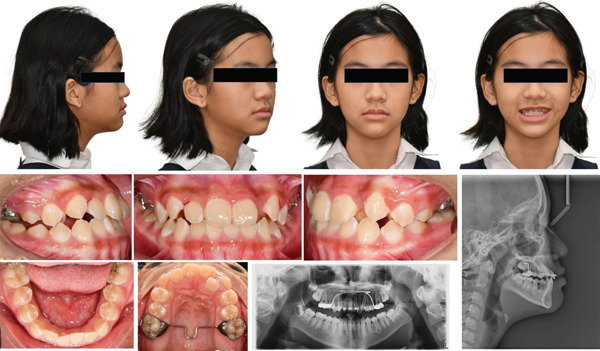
Postorthopaedic extraoral and intraoral photographs, panoramic radiograph and lateral cephalometric radiograph following completion of protraction facemask therapy.
